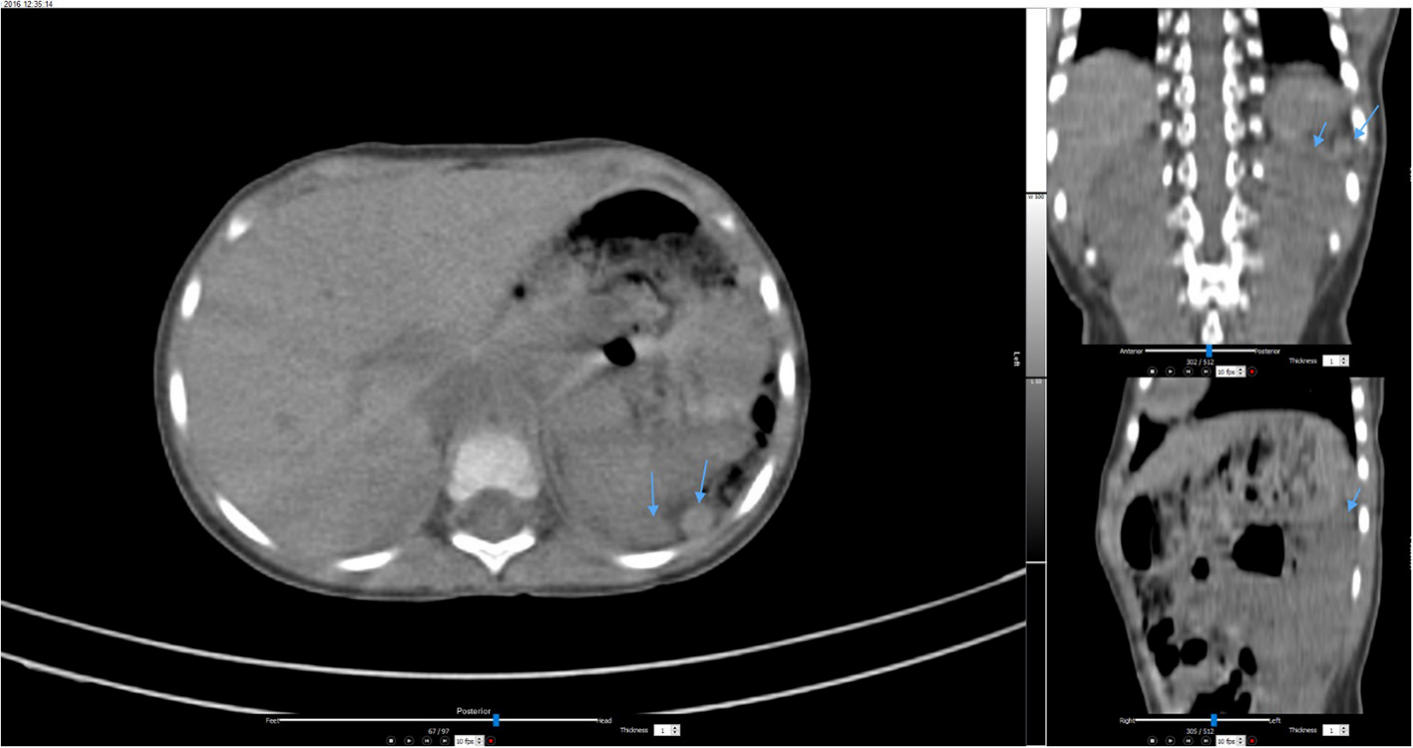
Single-photon emission computed tomography (SPECT-CT) images of the abdomen at 2 years of age showed two oval foci in the left upper abdomen (arrows) indicating splenunculi/splenules in the splenic bed.
